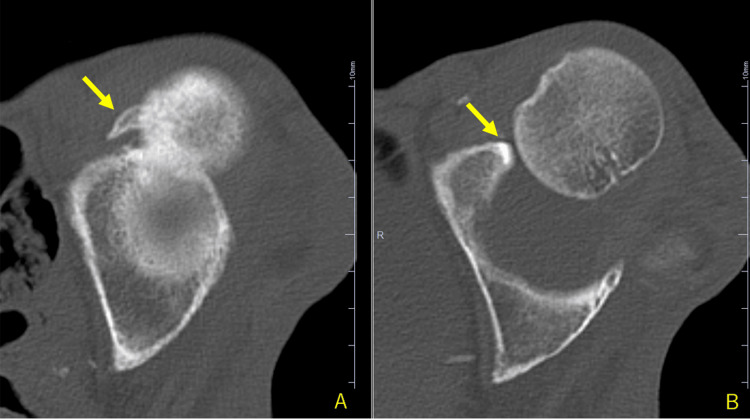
First CT scan of the left hip joint. A beak-shaped osteophyte is visible on the posterior side of the femoral head (A), along with bone wear on the anterior rim of the acetabulum (B).
